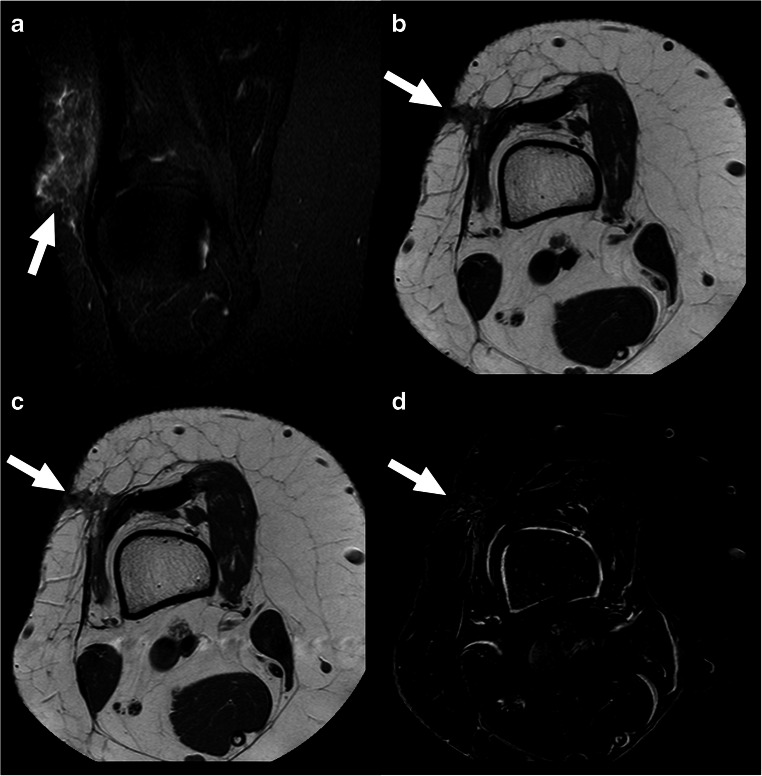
Example of false-negative MRI scan in a 21-year-old woman with synovial sarcoma who had undergone a Whoops procedure at the lateral side of the distal upper right leg. Coronal fat-suppressed T2-weighted (a), axial T1-weighted (b), gadolinium chelate-enhanced T1-weighted (c), and subtraction gadolinium chelate-enhanced (d) images at the level of the of the distal right upper leg. There are superficial soft-tissue changes at the lateral side of the distal upper right leg (arrows), without a contrast-enhancing nodular lesion or mass, which was interpreted as postsurgical changes without residual tumor. However, pathological examination after second surgery showed residual synovial sarcoma, extending beyond the superficial muscle fascia with muscle involvement
